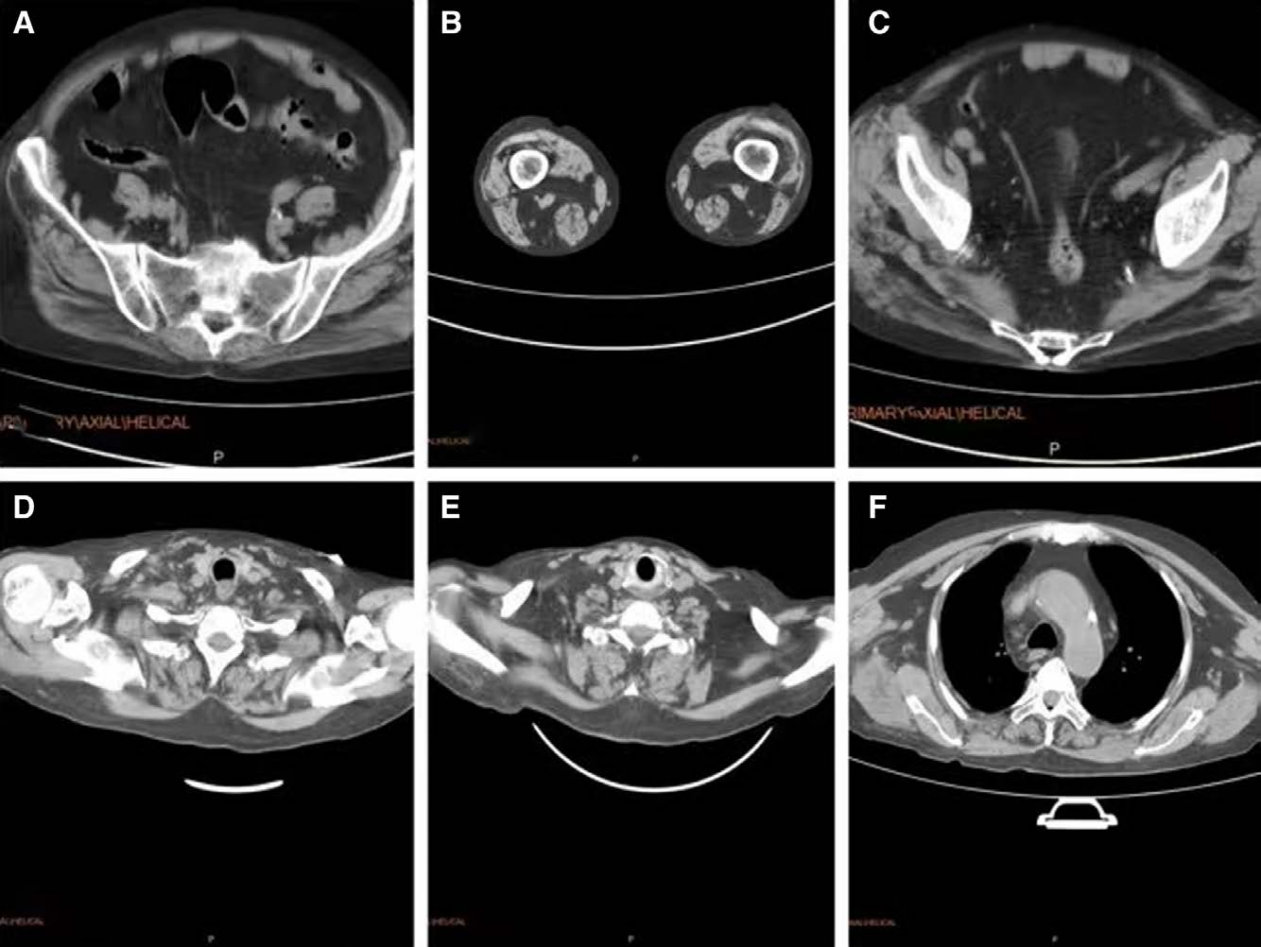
Computed tomography scan showed increased fat density shadows in the gluteus maximus, gluteus medius, and erector spinalis muscles (A), muscle atrophy, widened muscle spaces, and increased fat density shadows in the biceps femoris, intermediate femoris, and medial femoris muscles (B and C), increased fat density shadows in the levator scapula and trapezius muscles (D and E), and volume reduction, muscle atrophy, and increased fat density in the latissimus dorsi, scapula, pectoralis major, and pectoralis minor muscles (F).
